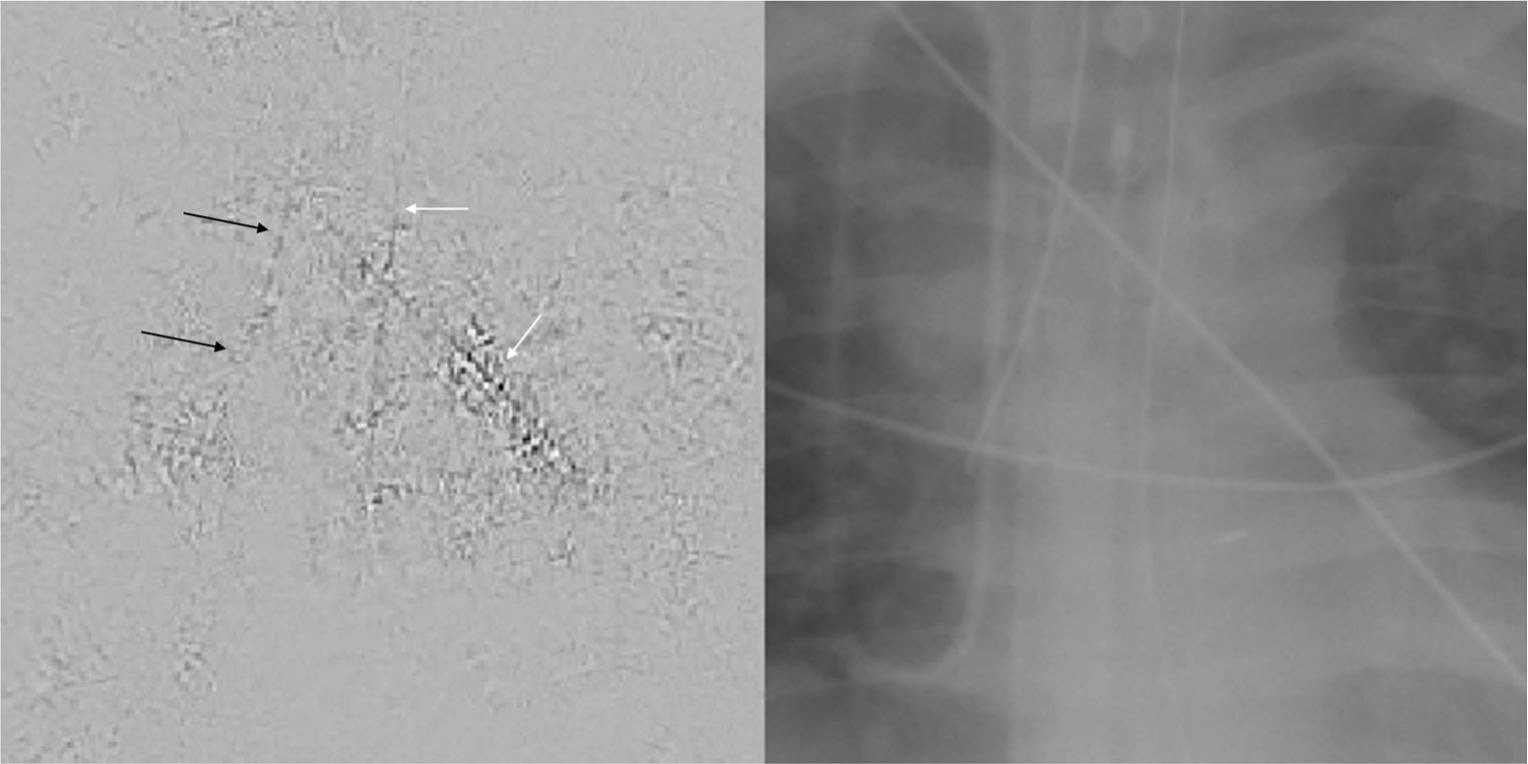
Saliency map on the *left* with corresponding radiograph on the *right* with low position of ET tube in the right main stem bronchus. On the saliency map, the *black arrows* point to the location of the ET tube. The *white arrows* point to an unrelated overlying catheter and an enteric tube. From the saliency map, the ET tube, enteric tube, and overlying catheter all have contributions to the prediction class, indicating that the network is not as well formed and is inferring from parts of the image (enteric tube and overlying catheter) that are not relevant to the prediction label (ET tube is low). This may explain the lower accuracy of this network compared to the others
